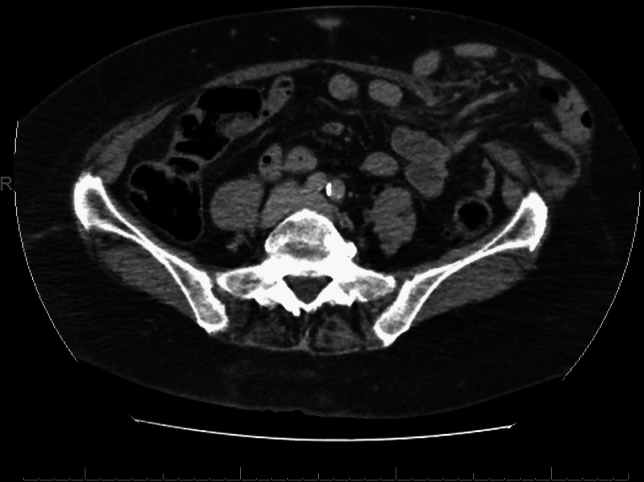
CT-Scan of left spigelian Hernia (Foto © U. Volmer)
